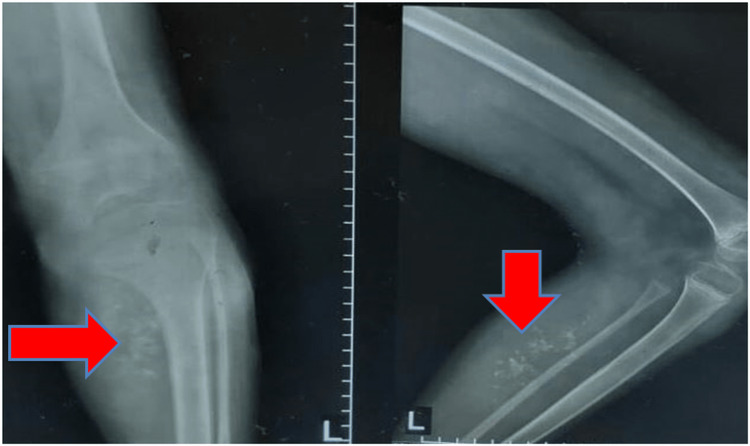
X-ray anteroposterior and lateral view of the left knee joint with femur, tibia, and fibula showing a soft-tissue mass containing phleboliths with underlying bones showing mild osteopenic changes in the medial aspect of the proximal part of the leg.
